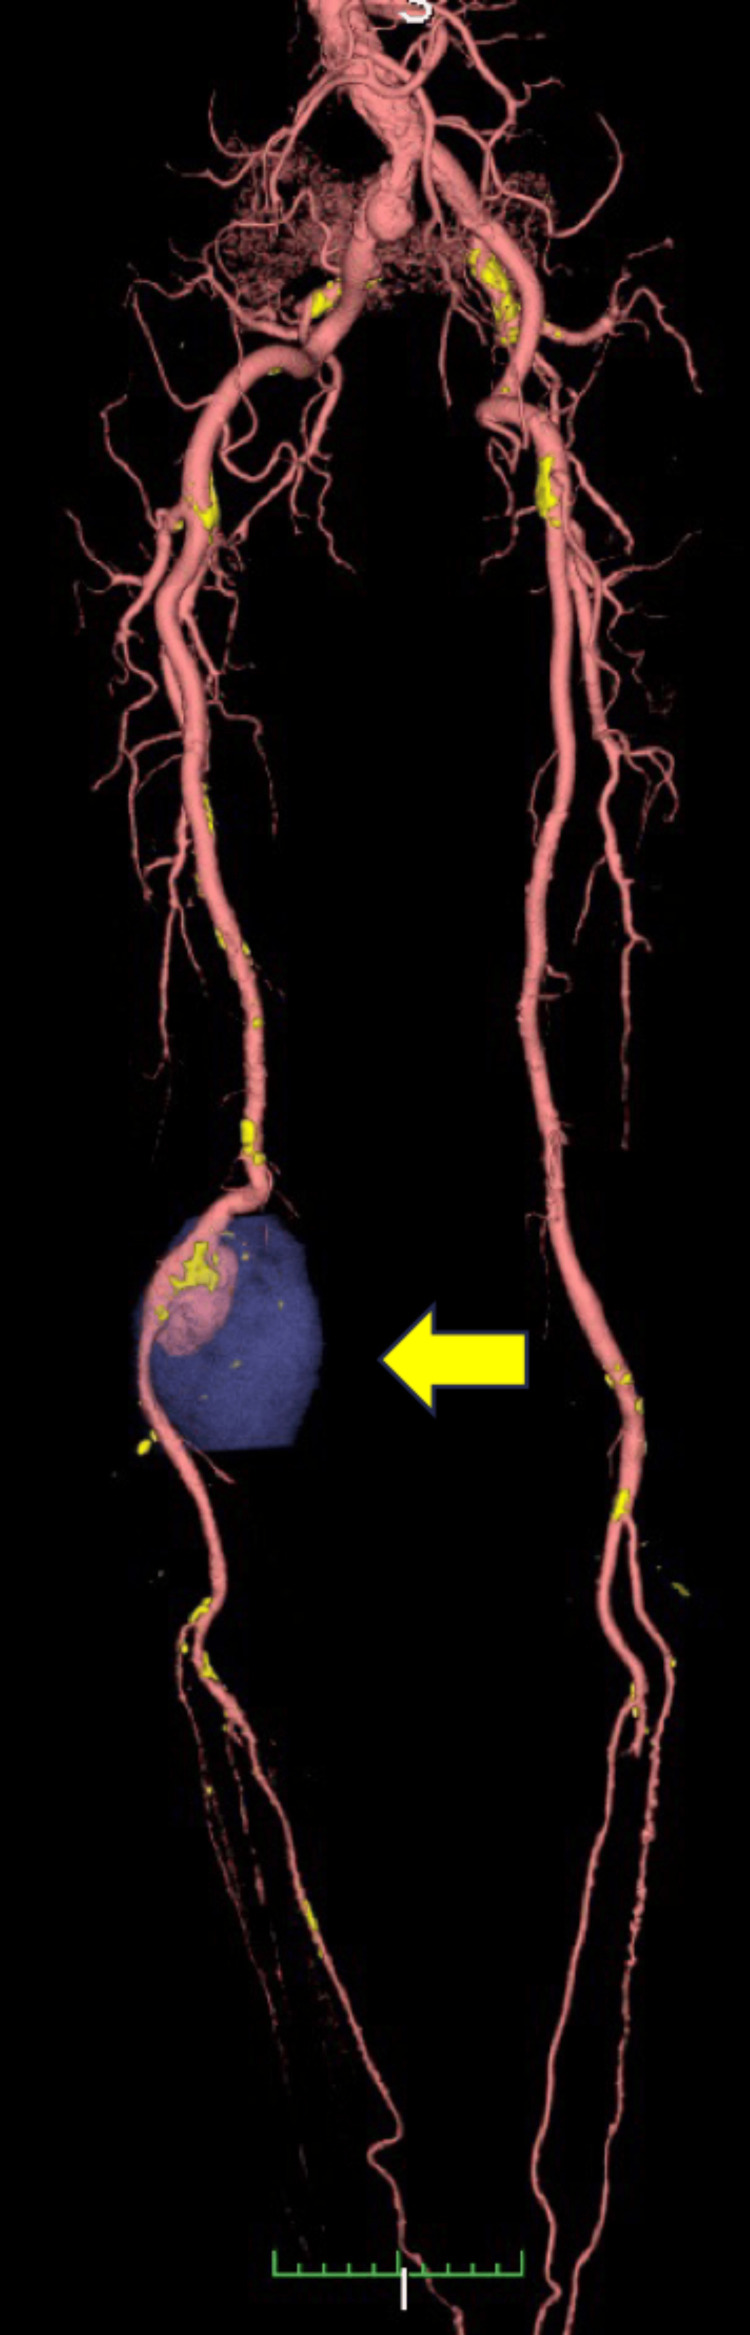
Preoperative 3D Computed tomography (CT) scan of arteries of the lower extremities This indicates that the aneurysm was saccular, with a maximum diameter of 34 mm × 24 mm (arrow), and that there was no blood flow into the aneurysm in part (blue-tined area).
